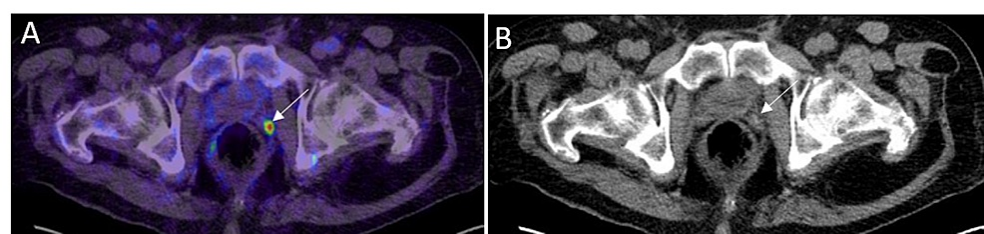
Regional lymph node metastasis A: Axial 68Ga PSMA PET/CT shows metastatic left periprostatic lymph node (white arrow). B: Axial CT shows the correlate small periprostatic lymph node (white arrow).
